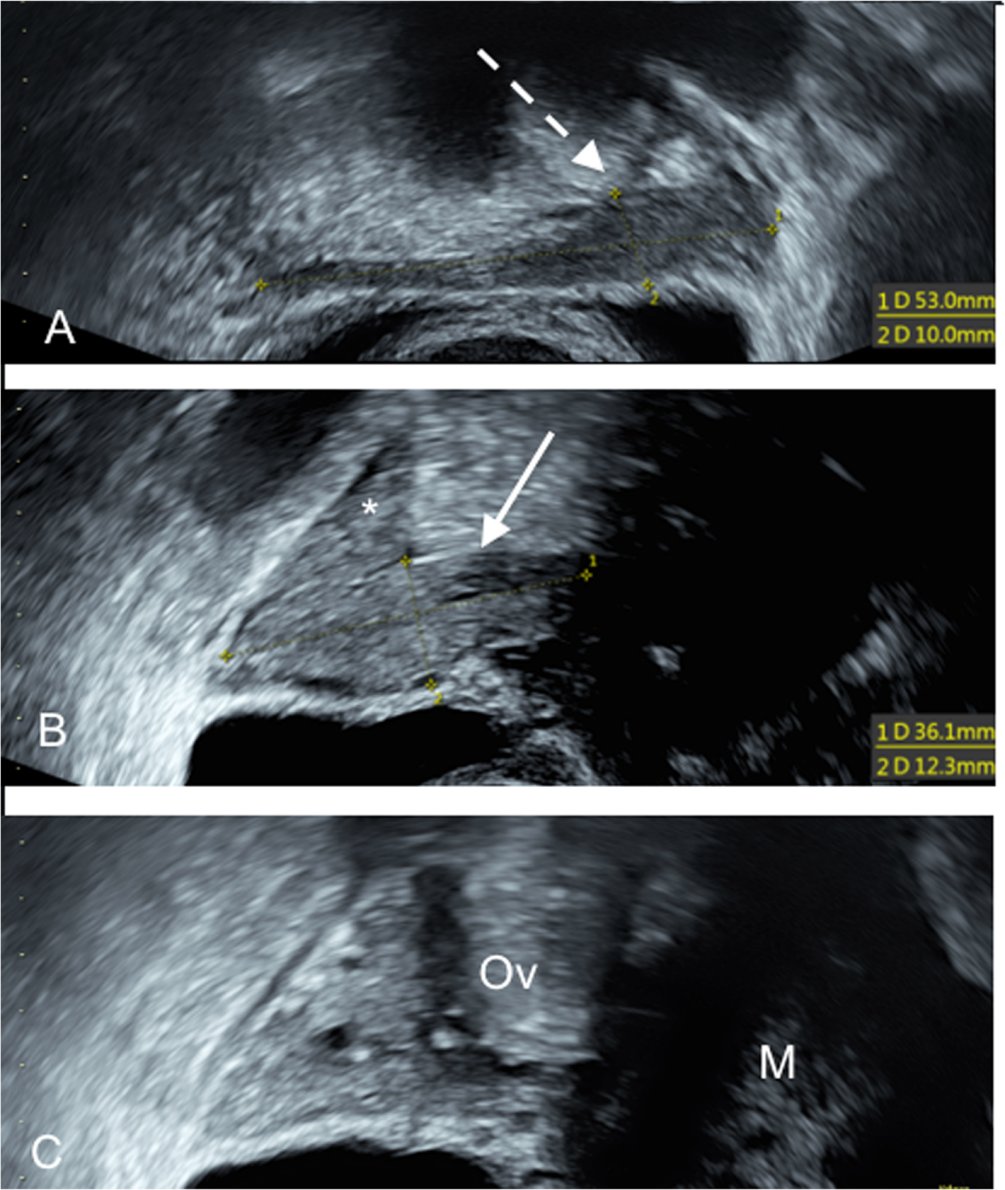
a A tear-shaped uterine remnant (dotted arrow) on the left side visualized by transvaginal ultrasound. b The right-sided uterine remnant (arrow) and the lower pole of ovary (asterixis) immediately above the uterine remnant. c Right ovary (Ov) and leiomyoma (M). (Voluson S10, GE Health, Chicago, Illinois, USA)
